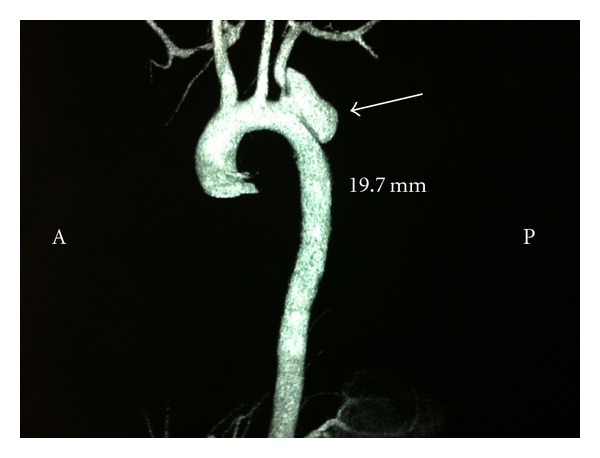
Angio-CT where we can appreciate the pseudoaneurysm (white arrow) which includes the origin of the left subclavian artery without affecting the left carotid artery.
